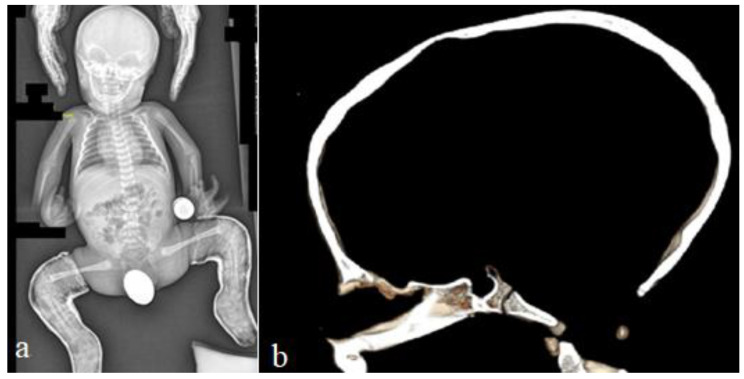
Skeletal survey; general skeletal radiograph at the age of one month showed multiple malformation complexes, starting from the skull and extending downwards to involve the upper and lower limbs, respectively. The skull showed apparent synostosis of the metopic suture. The upper limbs and lower limbs were notably associated with multiple dislocations (hips, wrists, knees, ankle and camptodactyly at the interphalangeal joints). The limb abnormalities were diagnostically important, in that there was a complete anterior dislocation of the tibia and fibula. Treatment was carried out using a plaster cast fixation of the hyperextended knee dislocation and clubfoot, due to the manipulation of the surgeon who, with one hand, held the knee in flexed position, shifting the tibia anterior and pushing the foot into the corrective position (a). 3D sagittal cranial CT scan showed craniosynostosis of the metopic suture associated with a well- formed ridge spreading from the metopic suture to involve entire sagittal suture, associated with the obliteration of the anterior fontanelle, with the eventual development of scaphocephaly (b).
